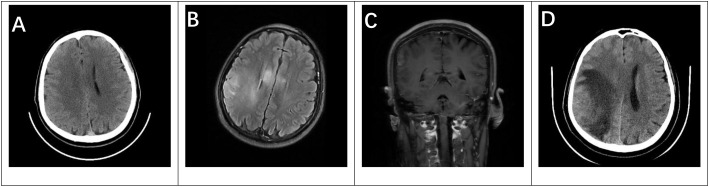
Patient’s cranial imaging findings from September 2024 (A–C) and February 2025 (D). (A) Cranial CT shows no significant mass effect. (B) T2 Flair hyperintensity observed in the right temporal-parietal lobe and corpus callosum. (C) Contrast-enhanced cranial MRI shows enhancement in the right temporal-parietal meninges and parenchyma. (D) Cranial CT reveals a distinct mass effect.
